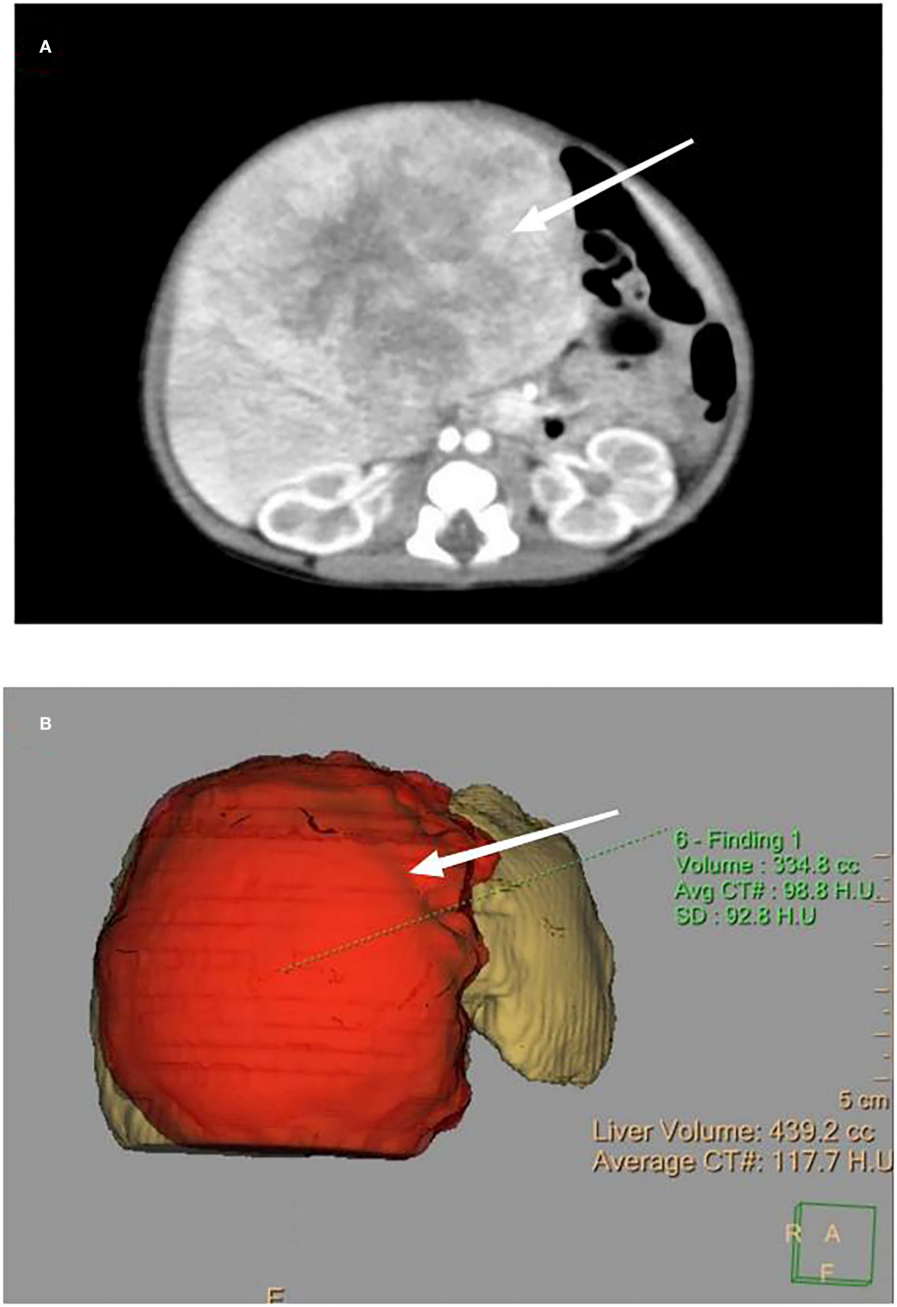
(A) Enhanced CT, as indicated by the arrow, showing a large, round mass with a size of 8.5 cm × 6.6 cm, a clear boundary, and uneven enhancement. (B) Three-dimensional model of the enhanced CT showing the appearance of the mass.
